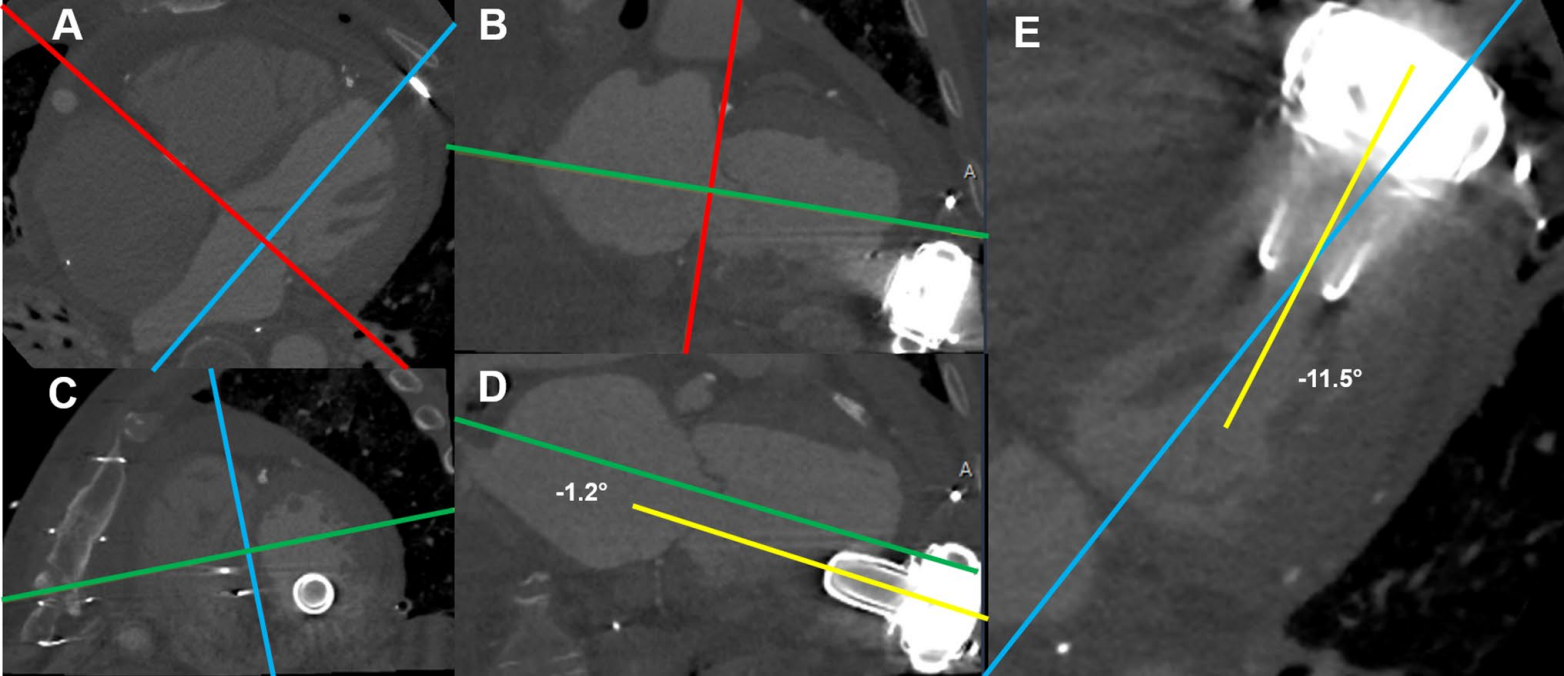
Example of Computed Tomography measurements. Computed tomography data were used to measure the implantation angle of the inflow cannula of the LVAD. Using a 3-dimensional MPR the LV long axis was reconstructed. A On a transversal plane, the MPR crosshair is aligned with the mitral valve annulus and the perpendicular part of the crosshair parallel to the LV long-axis. B This is repeated in the sagittal view to create a double oblique short-axis view of the LV. C The crosshair is further aligned to the septum to create a double oblique four-chamber and two-chamber view, with the crosshair parallel to the LV long-axis. (D + E) By adjusting the window settings the angle between the inflow cannula of the LVAD and the LV-axis can be visualized in the two-chamber (D; anterior or inferior) and four-chamber (E; septal or lateral) view. *LV* left ventricle, *LVAD* left ventricular assist device, *MPR* multiplanar reformation, *PT* pump thrombosis
